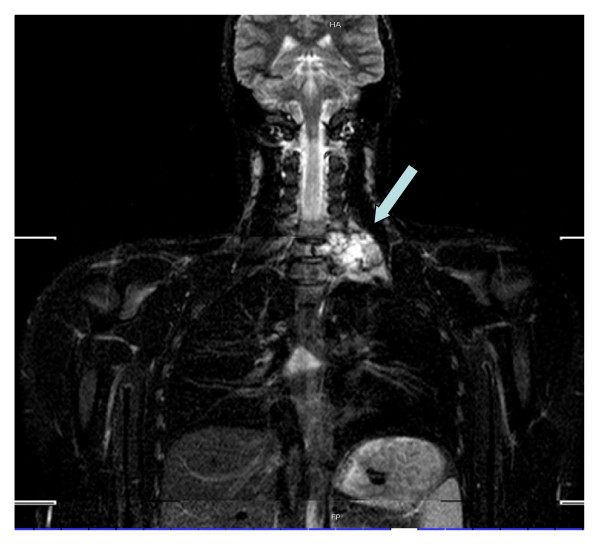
The coronal plane from the mobi-view MRI scan shows the expansiveness of the cyst (arrow).
